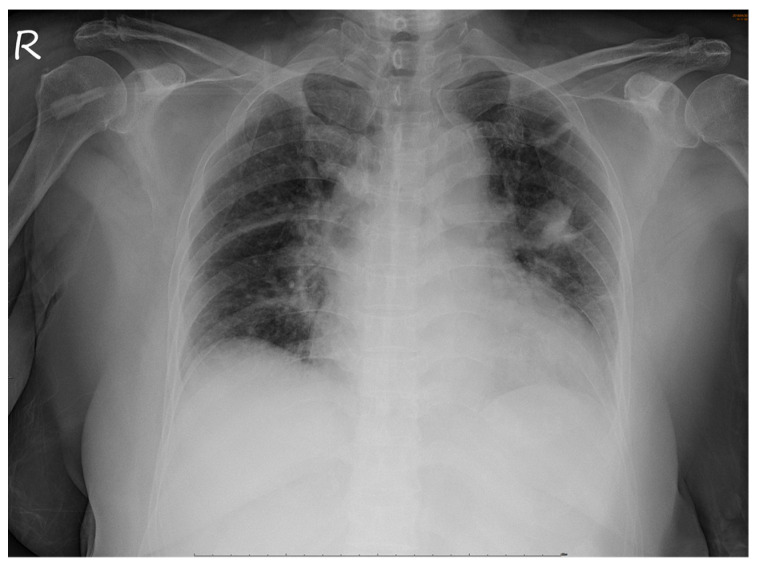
Chest radiograph. A non-homogenous patch is seen in the superior lobe of the left lung.
